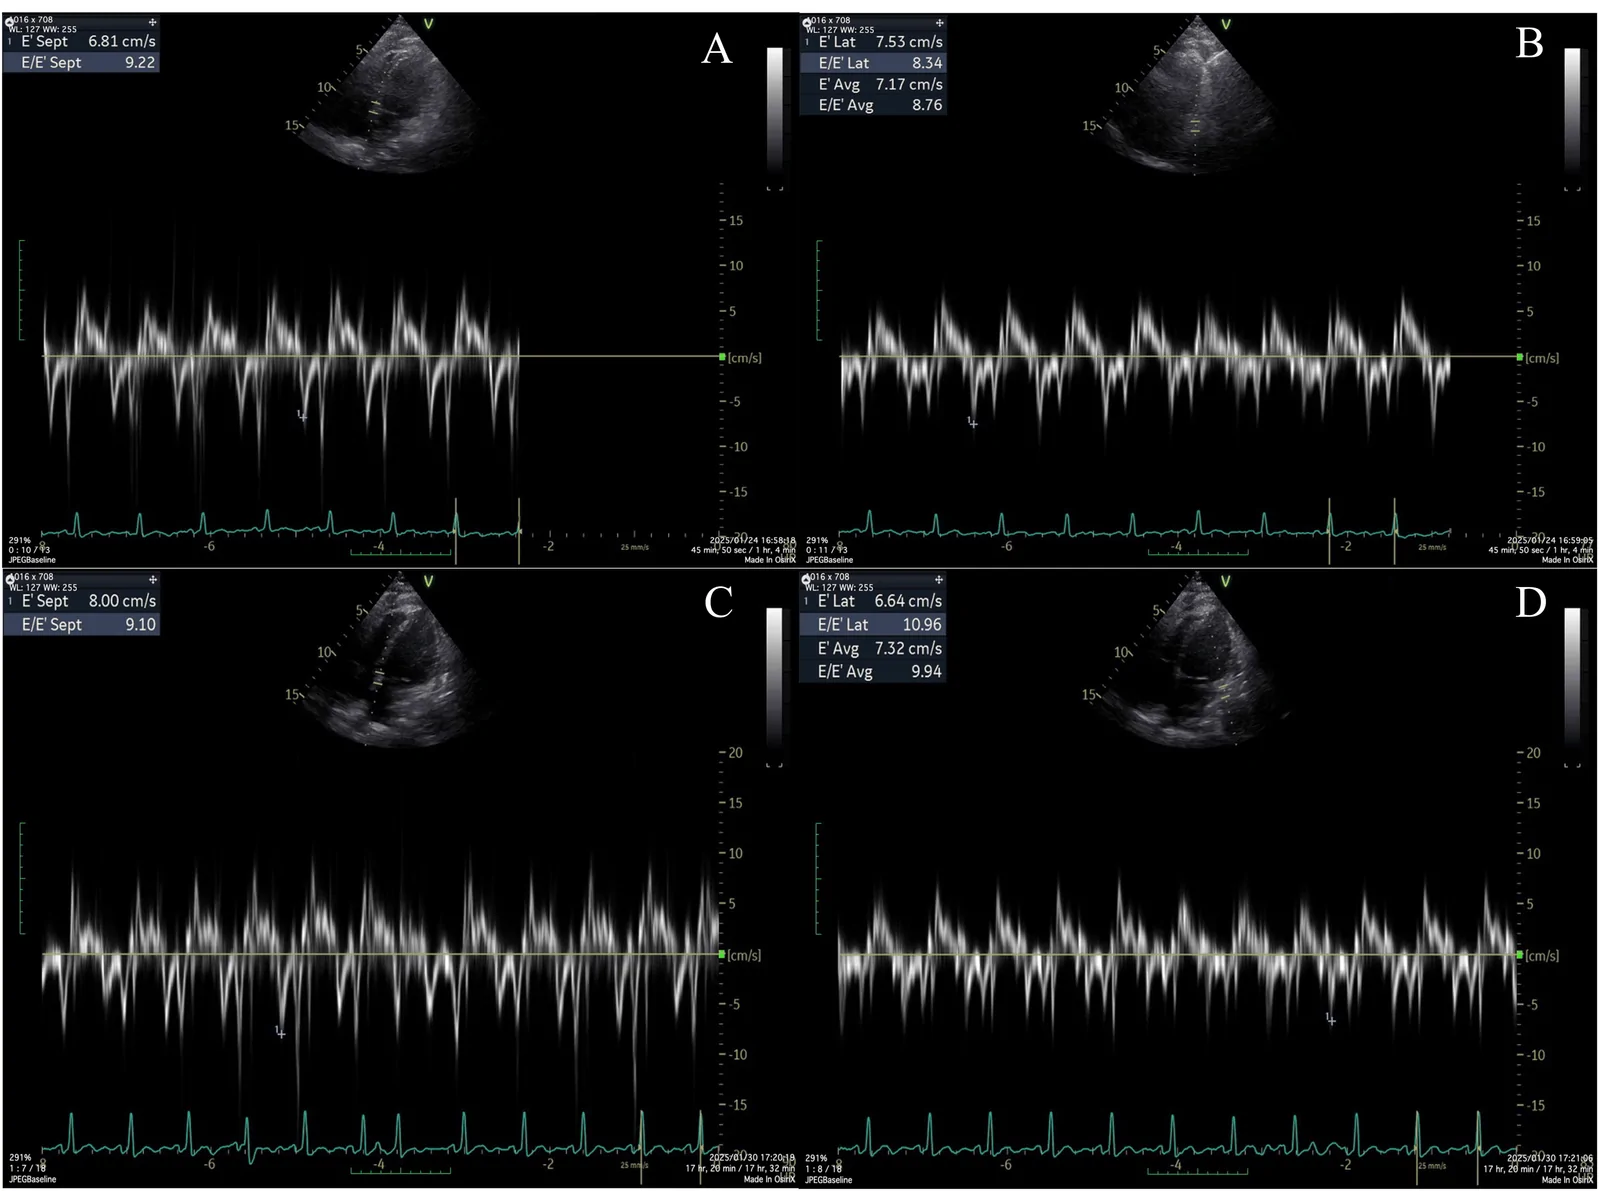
Transthoracic echocardiography on admission and on day 12 On admission (A and B), mitral annular velocities (e′) were 6.81 cm/s at the septal side and 7.53 cm/s at the lateral side. On day 12 (C and D), these values changed to 8.00 cm/s at the septal side and 6.64 cm/s at the lateral side, demonstrating that septal e′ > lateral e′ and indicating constrictive physiology.
